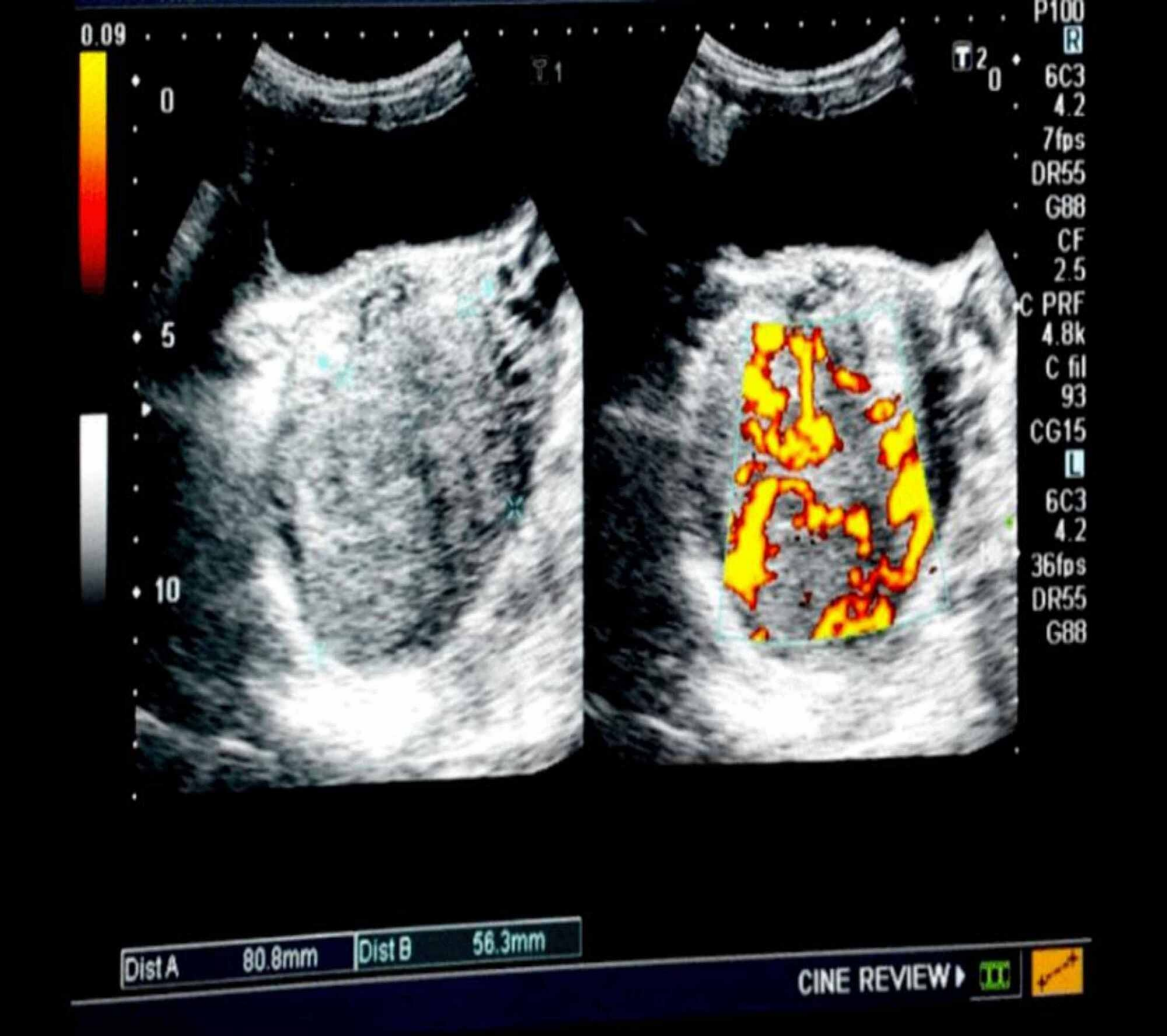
Pelvic ultrasound showing an echogenic mass in the left adnexal region. Doppler shows the mass to be highly vascular
